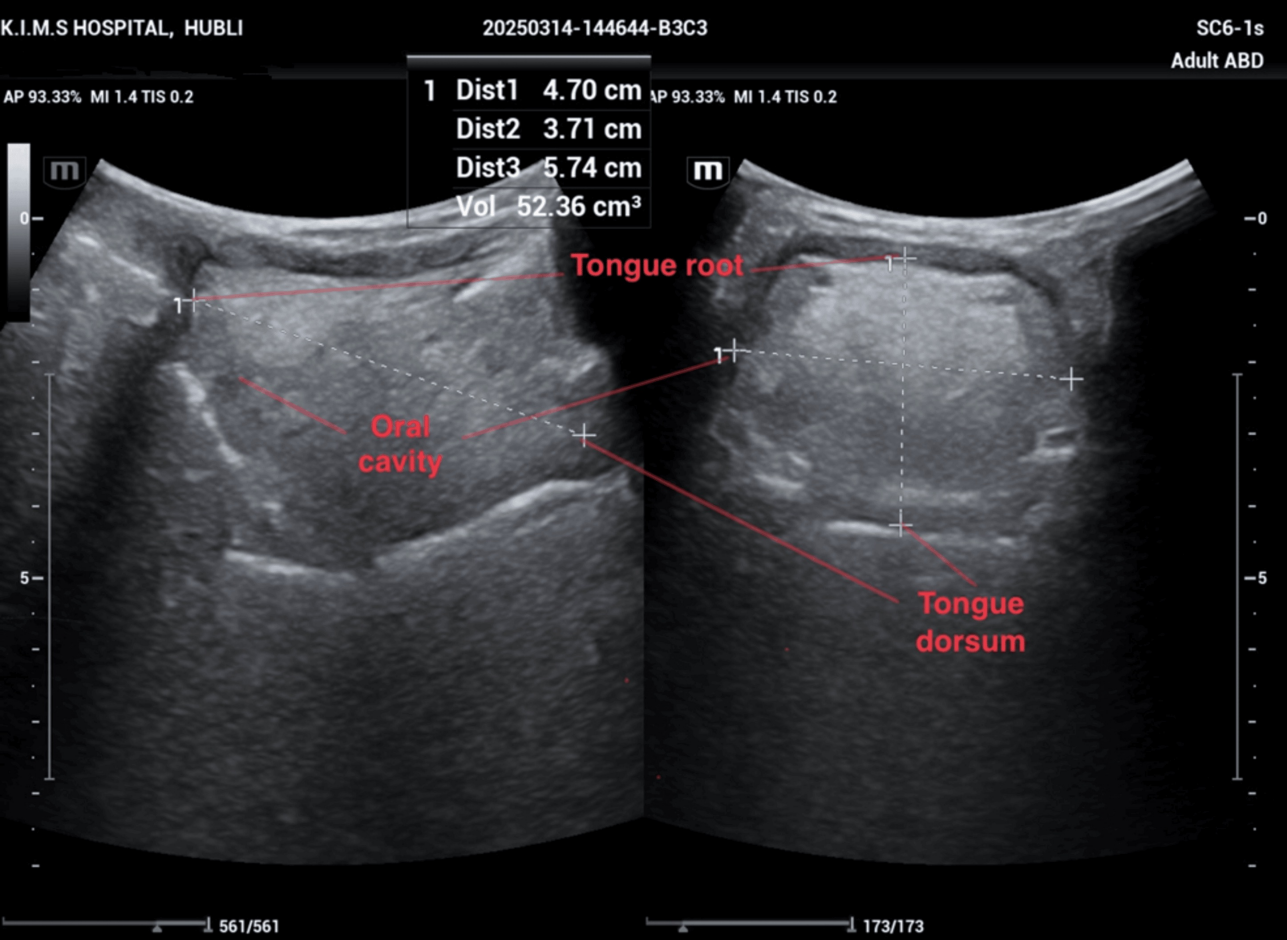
Ultrasonographic measurement of the tongue volume
